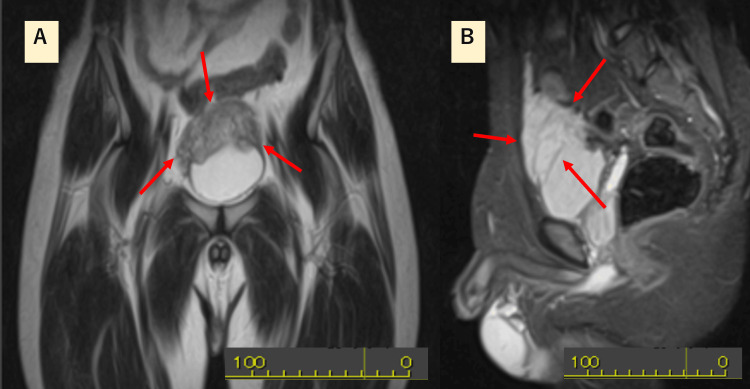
MRI images (A) Coronal pelvic T2-weighted short-tau inversion recovery (T2-STIR) image showing a slightly hypointense, heterogeneous mass; (B) Sagittal pelvic STIR image demonstrating a homogeneously hyperintense mass.
